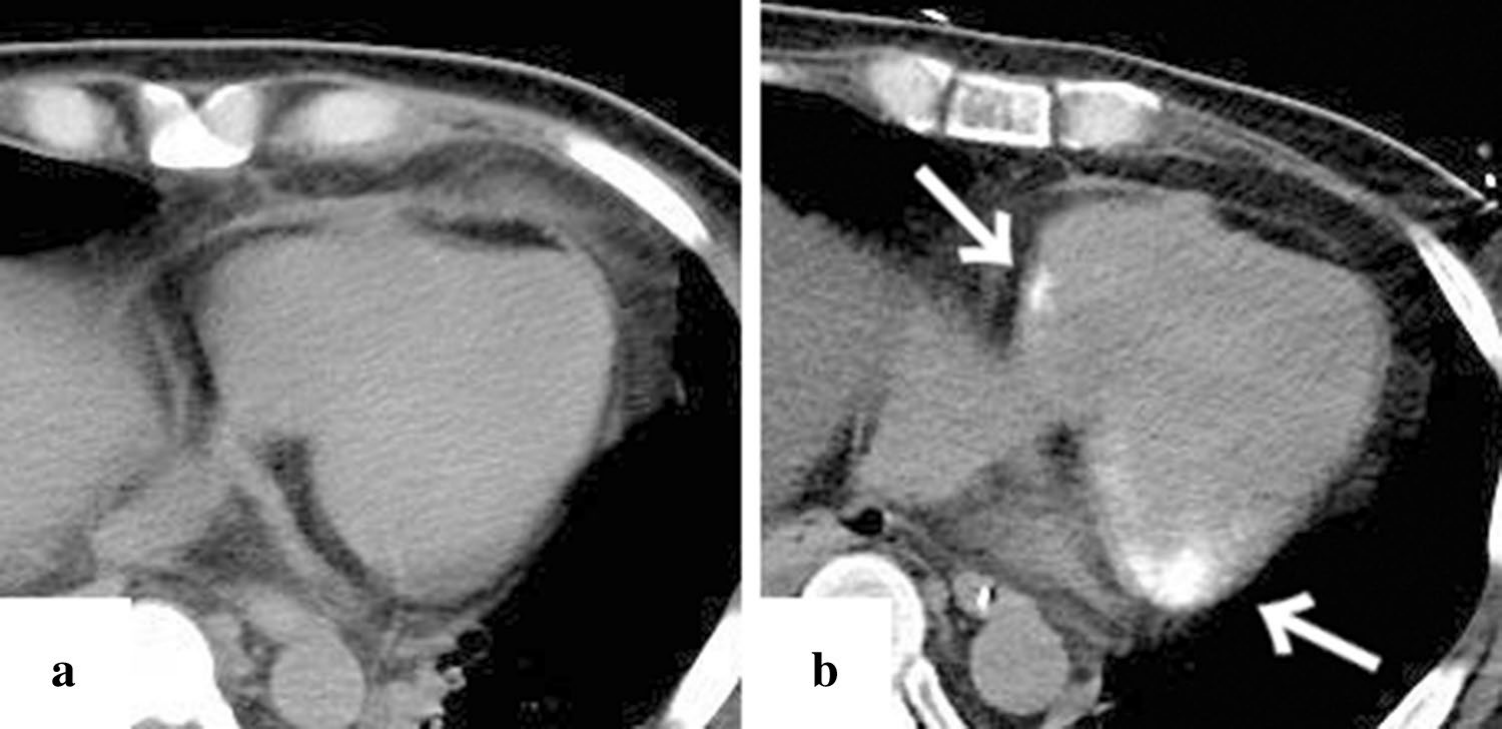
Representative case of myocardial calcification in a 51-year-old patient (case no. 13). Computed tomography (CT) imaging on day 1 since the onset of symptoms (a) and after one month of the admission (b). Calcification occurred in the myocardium (b, white arrow), and there was no calcification in the coronary arteries
